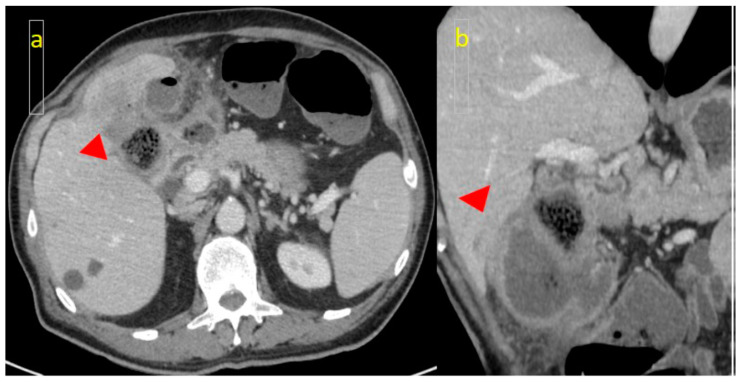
(a) CT scan: hypodense area (arrowhead) of the pericholecystic liver parenchyma, suggestive of invasion; (b) Coronal CT slice.
